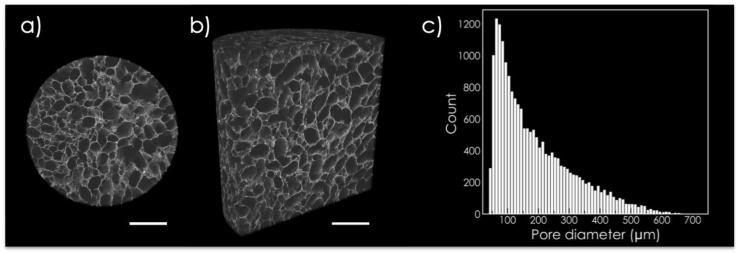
Structural characterization of recombinant collagen in the presence of magnesium (RCP.MgAp) scaffold (diameter = 4 mm; height = 4 mm) by micro-computed tomography. (a) Transversal and (b) longitudinal section of the scaffold. Scale bar=1 mm. (c) Graph bar displays pore size distribution estimated with ImageJ software.
